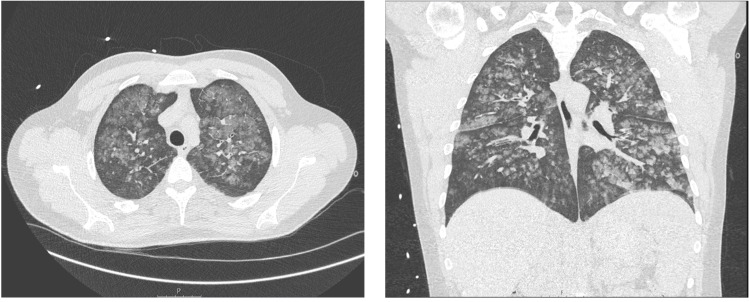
Thoracic CT scan on admission. Left: axial view. Right: coronal view
